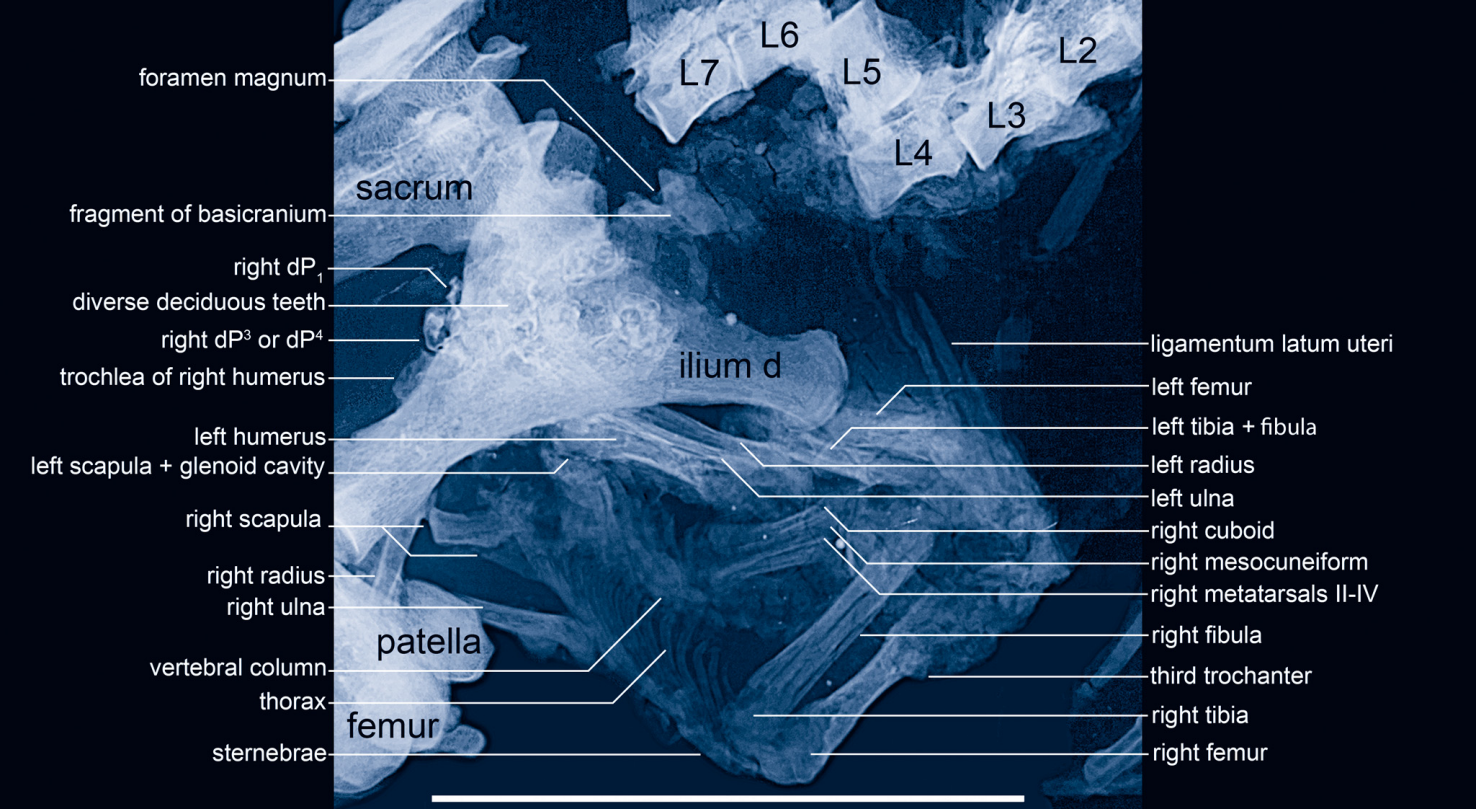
The fetus analyzed in detail by high-resolution micro-x-ray. Bones of the mare are indicated by black lettering, bones and teeth of the fetus by white lettering. L2-7 = lumbar vertebrae 2–7 of the mare. Scale = 10 cm.–Anatomical interpretation: Jens Lorenz Franzen; micro-x-ray: Senckenberg Forschungsinstitut Frankfurt, Jörg Habersetzer.
